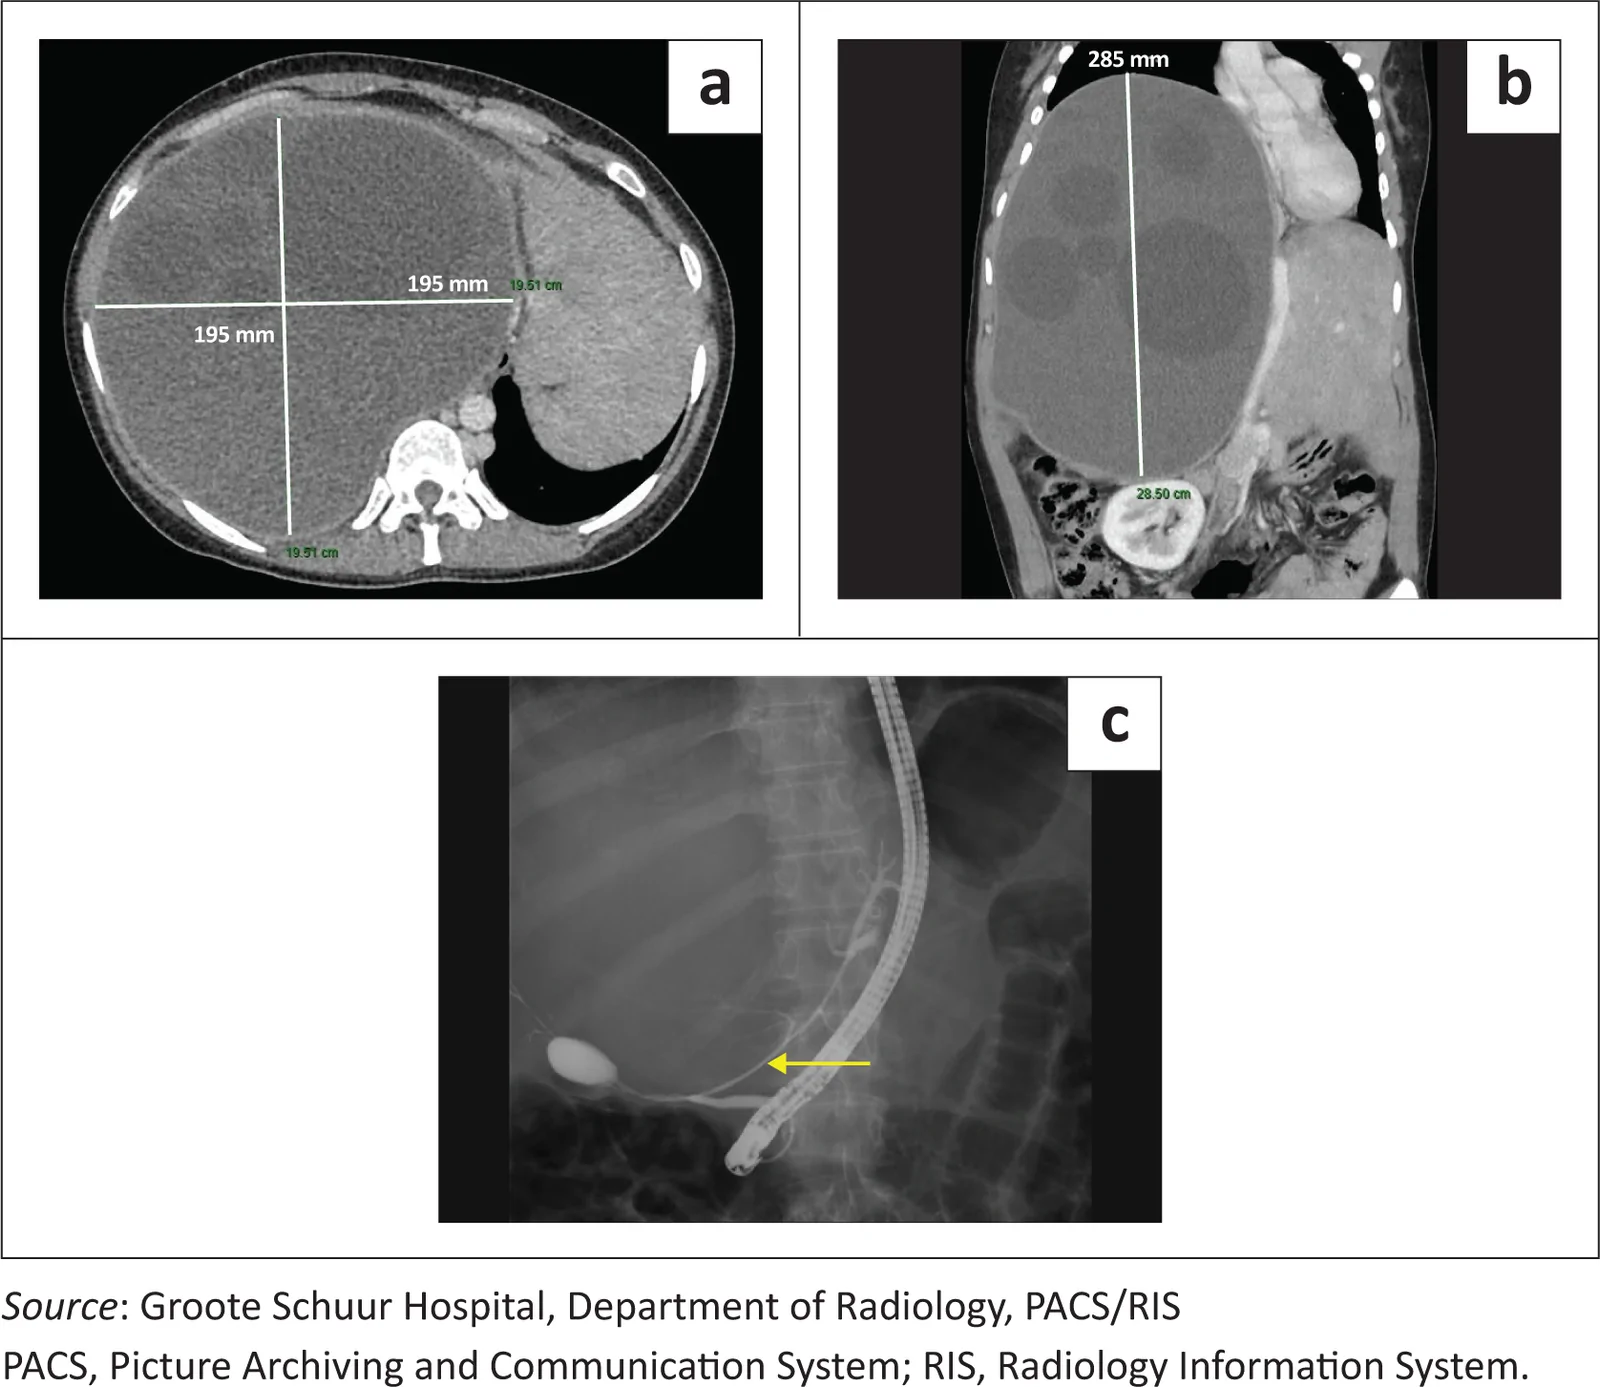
A CE-CT scan of a large cyst of the right hemi-liver measuring 195x195x285 mm (anteroposterior, transverse, craniocaudal) (a and b), in an HIV-positive patient female 29-years of age, compresses the right lung, displaces the heart into the left hemithorax and displaces the right kidney inferior and medial. An endoscopic retrograde cholangiopancreatography (c) shows the common hepatic duct compressed and stretched over the cyst (arrow).
